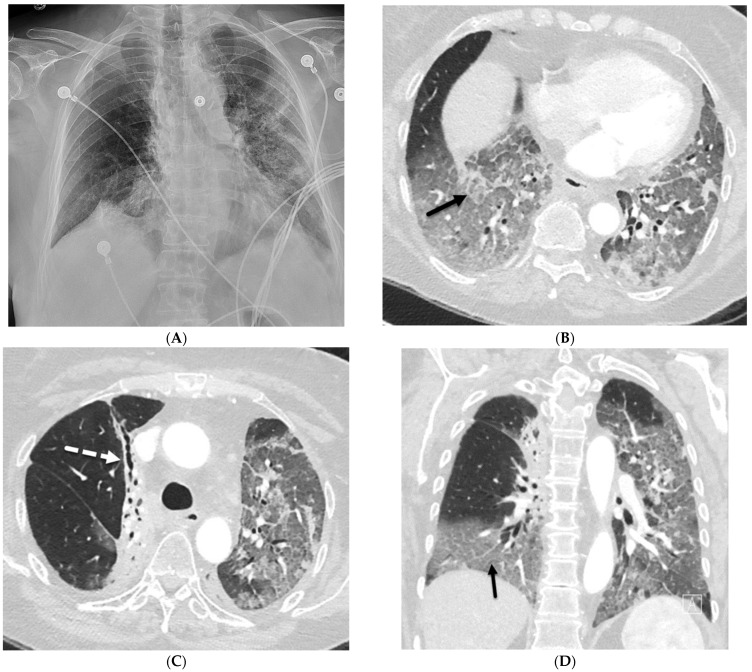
A 61-year-old female with a history of metastatic non-small-cell lung cancer presents with acute hypoxic respiratory failure due to COVID-19 pneumonia. A frontal chest radiograph (A) demonstrates interstitial prominence in the left lung. Axial (B,C) and coronal (D) chest CT images demonstrate radiation fibrosis in the right perihilar region (dashed white arrow). Diffuse ground glass opacities are noted throughout the left lung and right lung base with interlobular septal thickening (solid black arrows).
